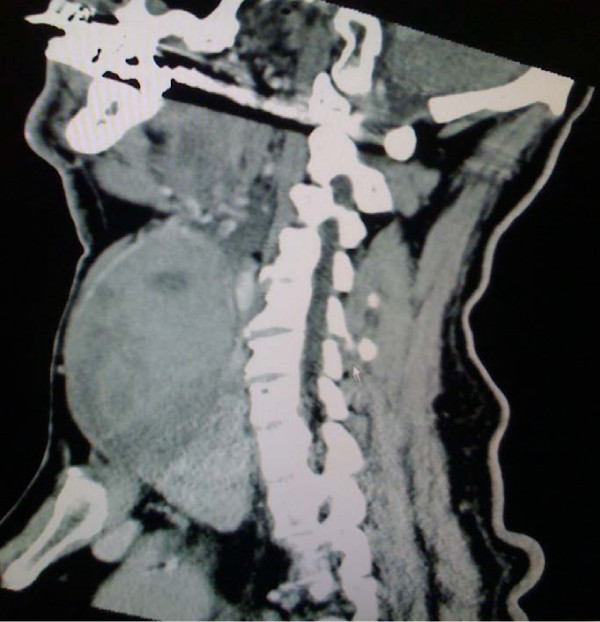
Sagittal computed tomography (CT) image showing the extent of the mass and the contrast enhancement in its caudal region.
